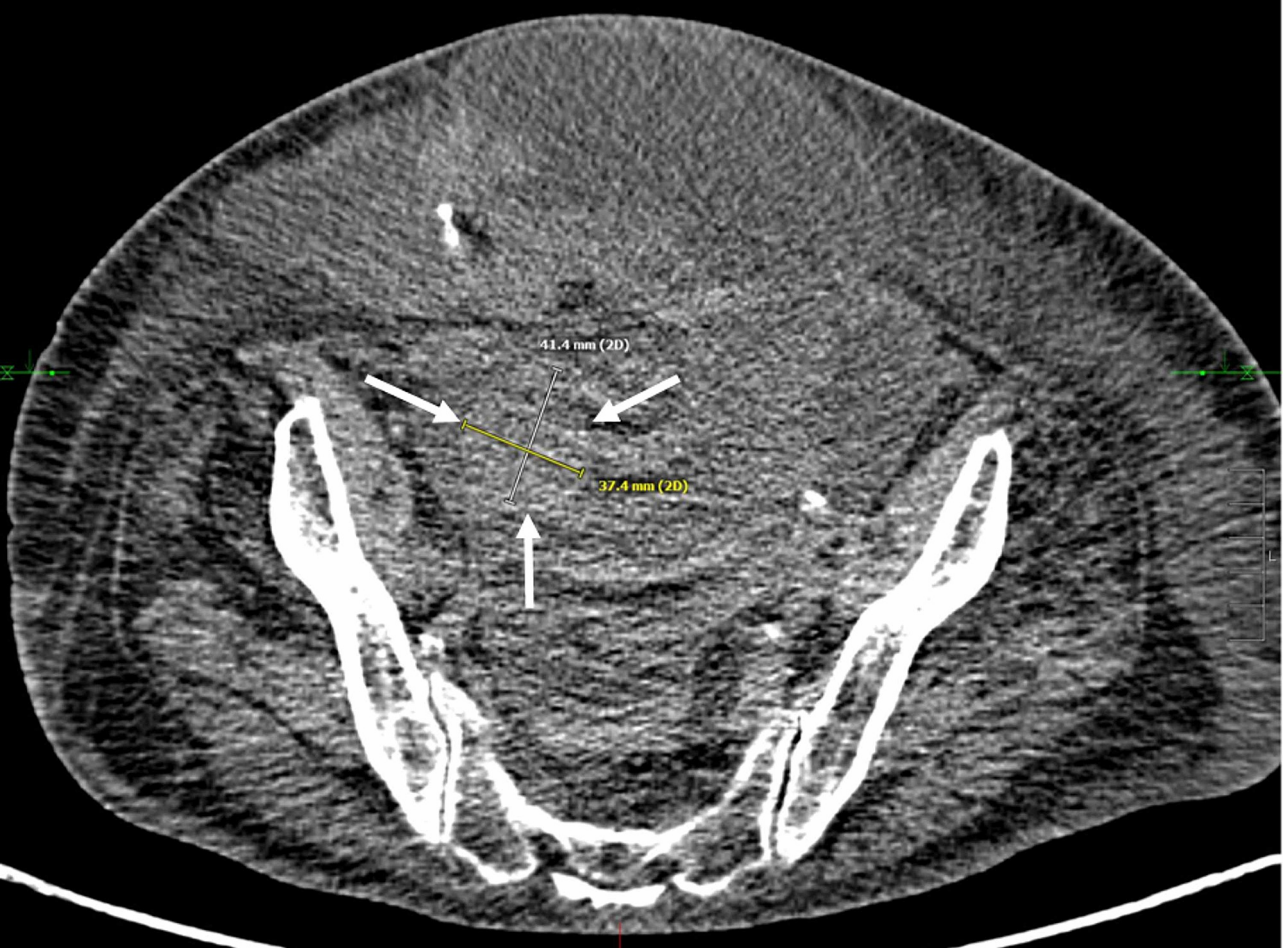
Surveillance CT nine months following cryoablation of the right ovarian mass Axial non-contrast CT obtained nine months post-ablation demonstrates decreased size of right adnexal mass (white arrows) measuring 4.1 cm in greatest dimension.
